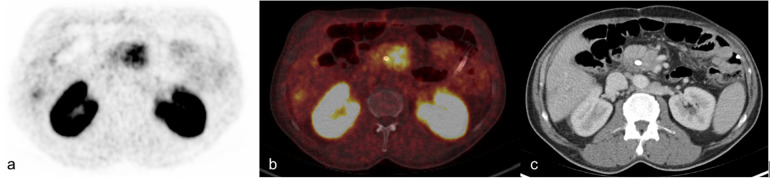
Primary staging of 59-year-old man with pancreatic cancer and simultaneous liver metastases (139 MBq ^68^Ga-trivehexin, 61 min p.i). (a) PET image (b) fused image with correlating ceCT, (c) contrast-enhanced CT in transversal plane.
